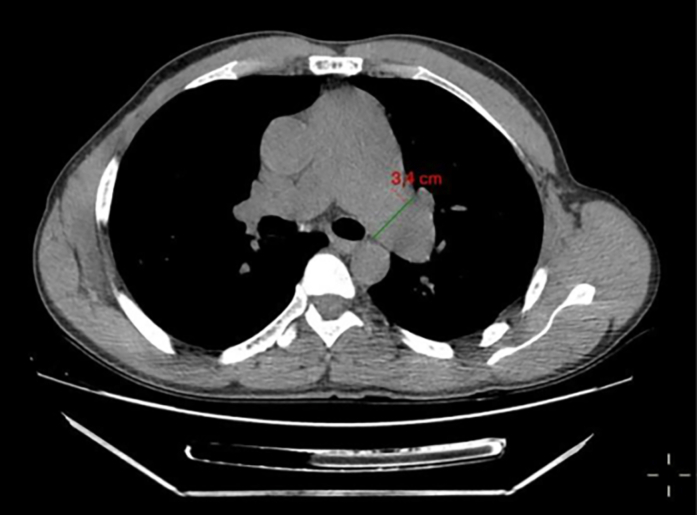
Chest Computed Tomography Scan Showing Left Pulmonary Artery Aneurysm With a 34-mm Diameter
